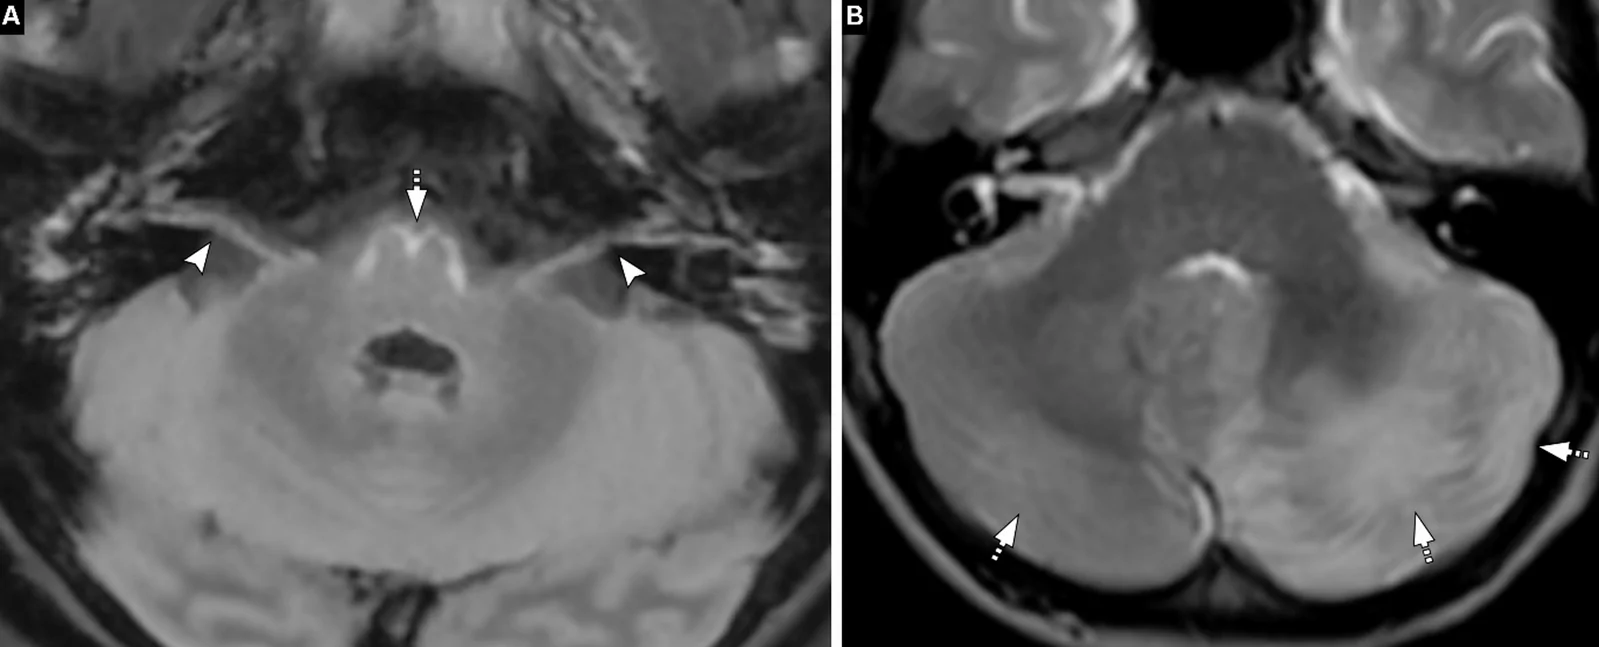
Meningitis and cranial nerve involvement. A A 45-year-old female with fever for 11 days, vomiting for 10 days, and bilateral lower-limb weakness for 2 days, diagnosed with scrub typhus, underwent an MRI of the brain. Axial post-contrast T2 FLAIR shows leptomeningeal thickening with enhancement along the brainstem (dotted white arrow), consistent with meningitis. Additionally, there is thickening and enhancement of bilateral vestibulocochlear nerve complexes (white arrowheads). Cerebellitis. B A 10-year-old female with fever, altered sensorium, and swaying, diagnosed with scrub typhus, underwent an MRI of the brain. Axial T2-weighted image shows increased signal in bilateral, left greater than right cerebellar foliae (dotted white arrows), consistent with cerebellitis
